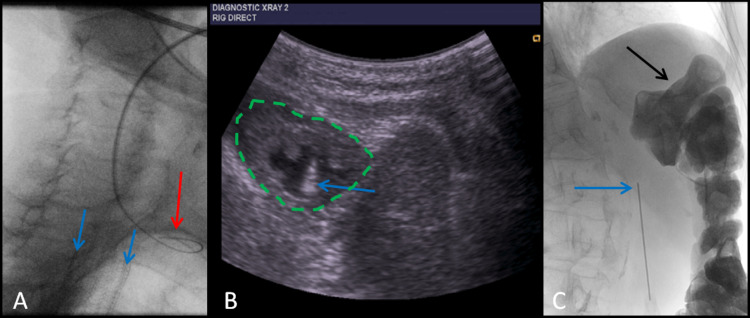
Oblique fluoroscopic images of the proximal esophagus (A), axial ultrasound of the stomach (B), and fluoroscopic image of the upper abdomen (C) A: Fluoroscopic image of failed oropharyngeal cannulation of esophagus showing coiling of the guidewire in the anterior oropharynx (red arrow). The upper borders of the tracheal stent are identified (blue arrows). B: Axial ultrasound image of the stomach (broken green line) showing successful ultrasound-guided puncture, and acoustic shadow from the Chiba needle within the stomach lumen (blue arrow). C: Fluoroscopic image of Chiba needle (blue arrow) after gastric puncture showing a paucity of air in the stomach prior to retrograde insufflation. The colon (black arrow) has been outlined by soluble contrast which was administered slowly over the preceding 48 hours.
